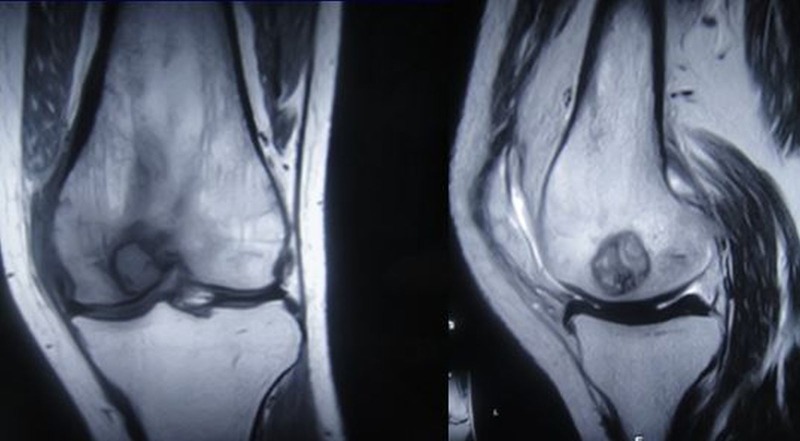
MRI showing increased intensity of the lesion
